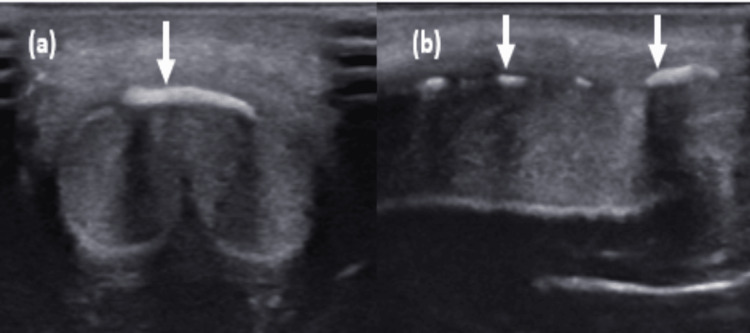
(a) Axial and (b) longitudinal B-mode ultrasound images showing linear calcific plaques along the dorsal aspect of the shaft of the penis
